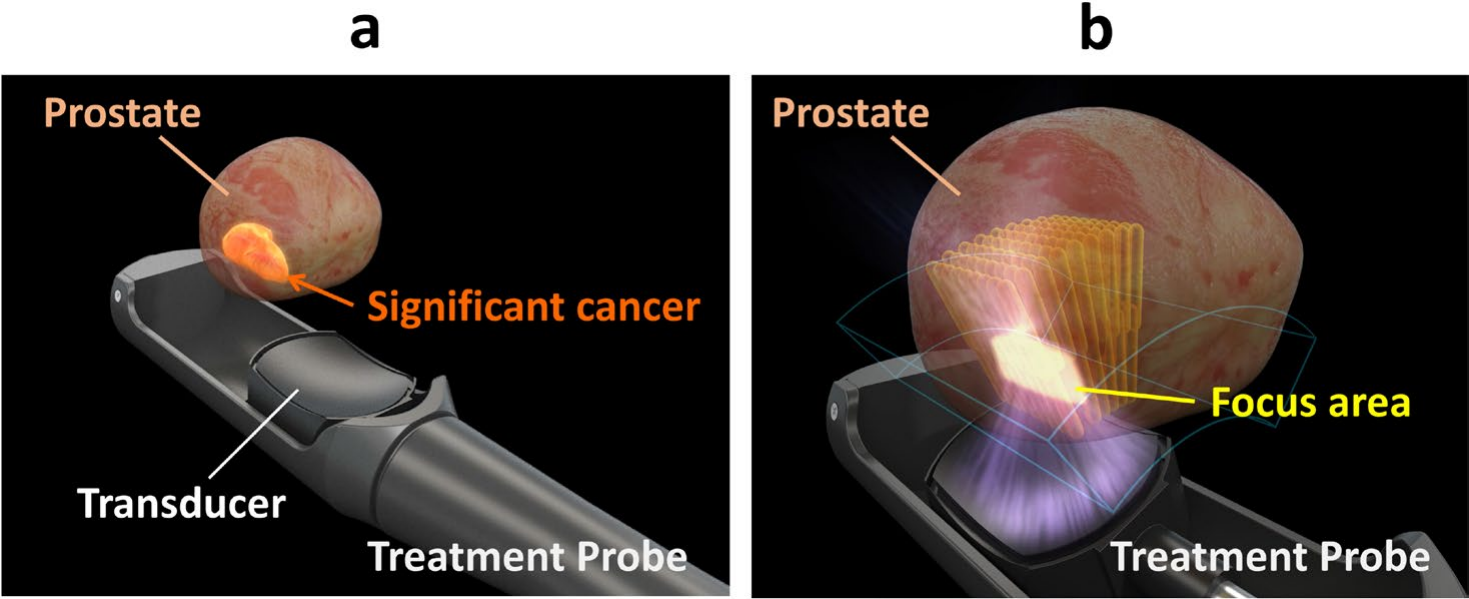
Computer graphic images of a commercial device for high‐intensity focused ultrasound for prostate cancer, Sonablate 500. In high‐intensity focused ultrasound (HIFU) treatment, a probe is inserted into the rectum, and the treatment area is planned using transrectal ultrasound imaging (a), which then emits ultrasound waves from a spherical transducer, delivering focused energy to millimeter‐sized target points, where its thermal and mechanical effects destroy the prostate tissue, with the focal areas moving in an overlapping pattern to cover the entire planned region (b). The image usage has been approved by Sonablate Corp.
